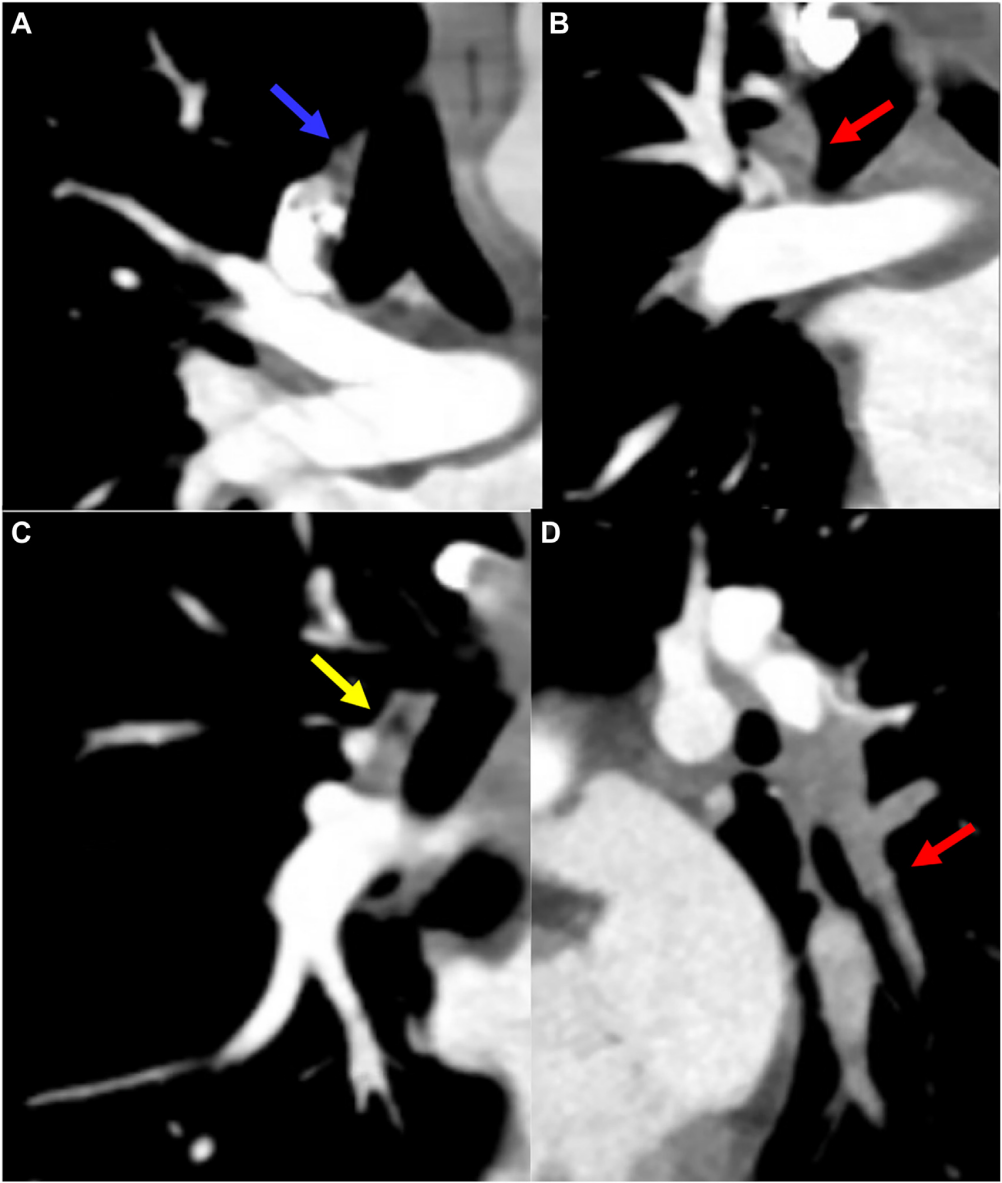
(a-c) Computed tomography angiography (axial view) showing increasing pulmonary emboli in the right lower segmental pulmonary arteries (PAs; blue arrow), extending into subsegmental PAs (red arrow), and new emboli in the right upper lobe PA (yellow arrow). (d) Filling defect in the left lower lobar PA, completely occluding the left lower lobe and lingular PAs (red arrow).
